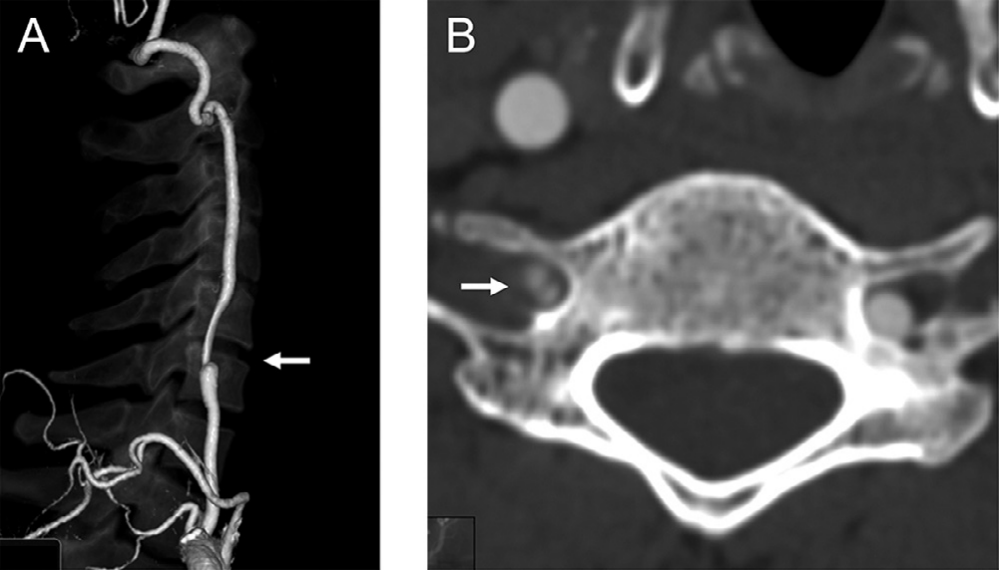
Extracranial vertebral artery dissection diagnosed by computed tomography angiography. Computed tomography angiography revealing the right extracranial vertebral artery dissection (arrow) (A). Axial image of computed tomography angiography demonstrating an intimal flap in the right extracranial vertebral artery (arrow) (B).
